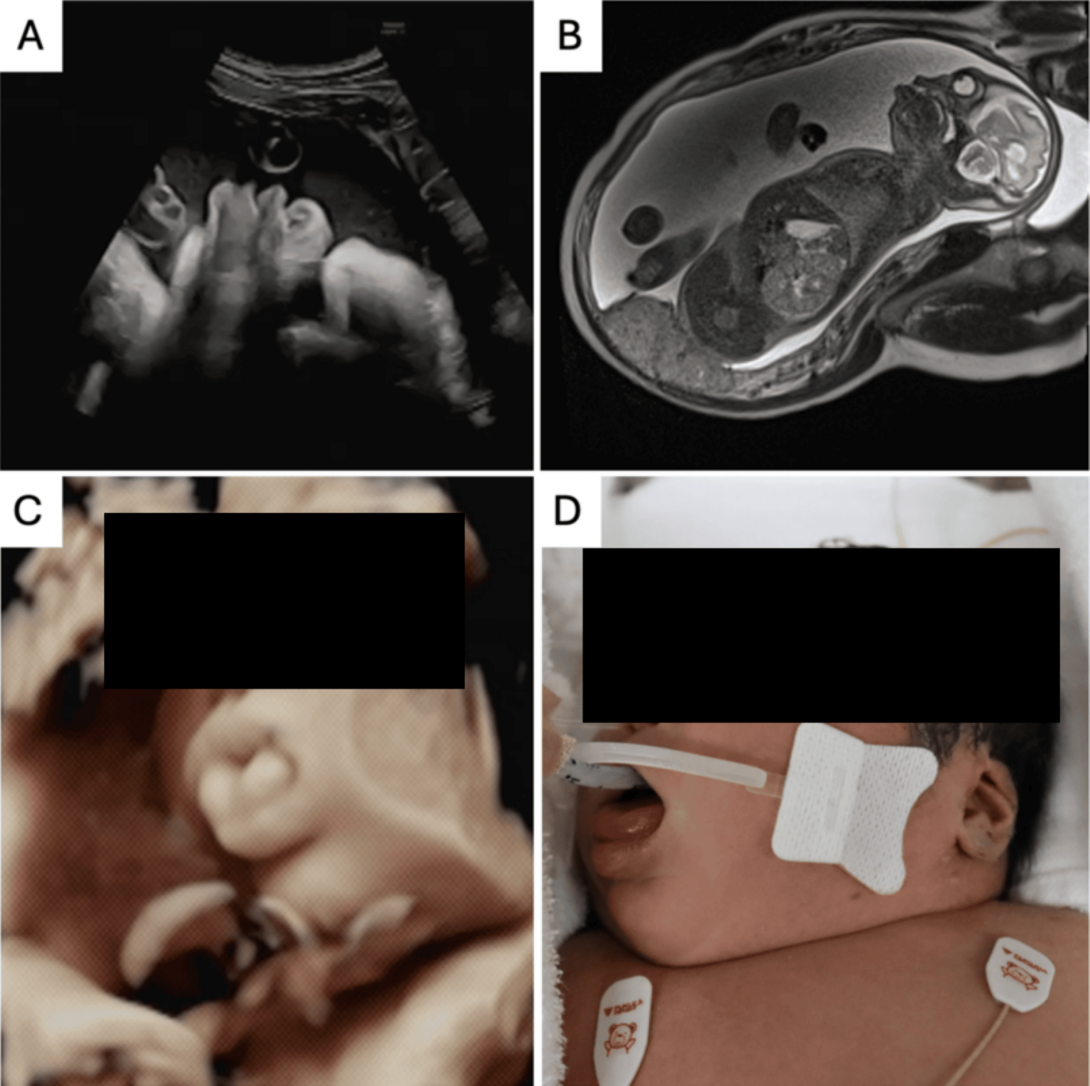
Perinatal findings of the fetus affected by BWS (A) Transabdominal ultrasound image at 31 weeks of gestation showing fetal macroglossia. (B) Fetal MRI at 31 weeks of gestation revealed bilateral nephromegaly, macroglossia, and polyhydramnios. (C) A 3D ultrasound image at 35 weeks of gestation demonstrated the fetal tongue protruding outside the oral cavity. (D) Postnatal photograph of the neonate showing macroglossia, consistent with the clinical feature of BWS. BWS, Beckwith-Wiedemann syndrome
